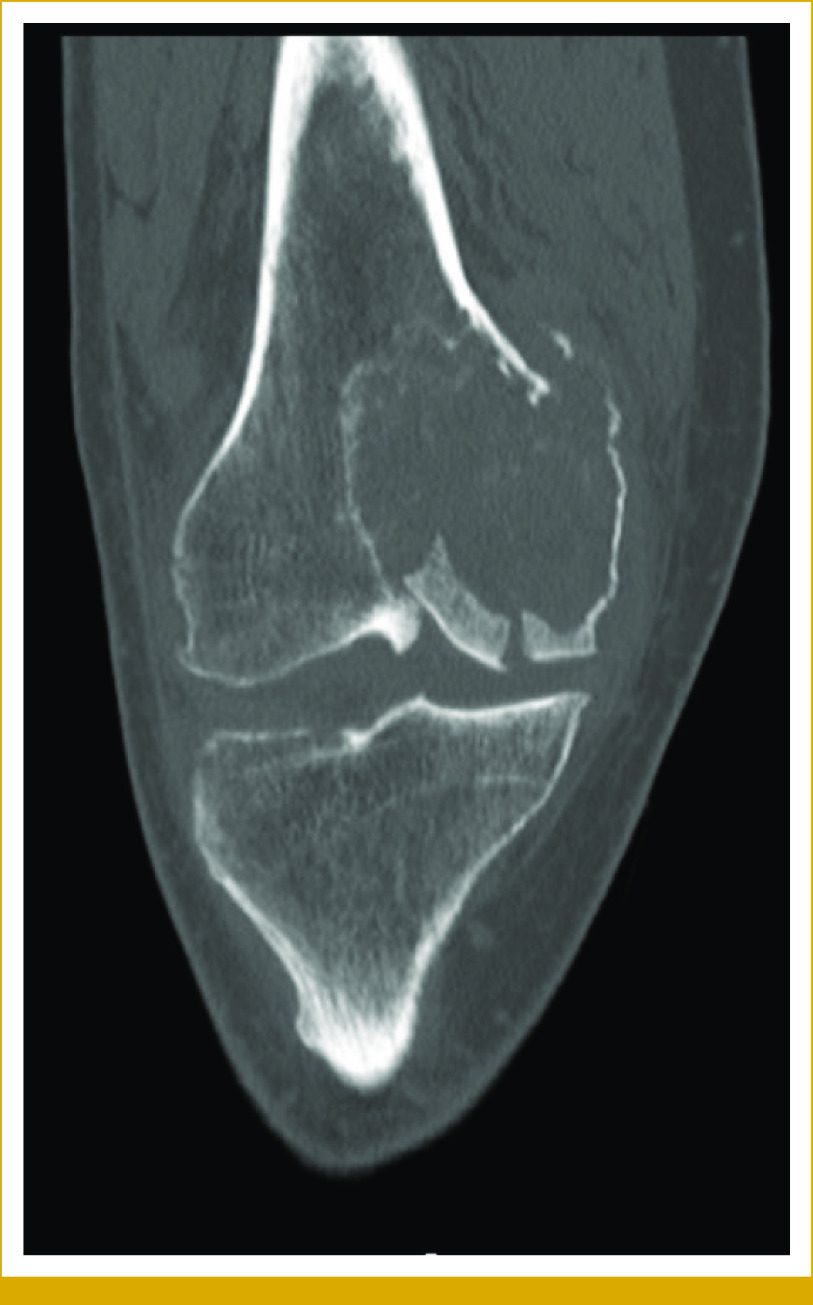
Coronal computed tomography image of a lytic lesion centered in the medial femoral condyle corresponding to the primary osteosarcoma at presentation. Note the presence of a pathologic fracture.
